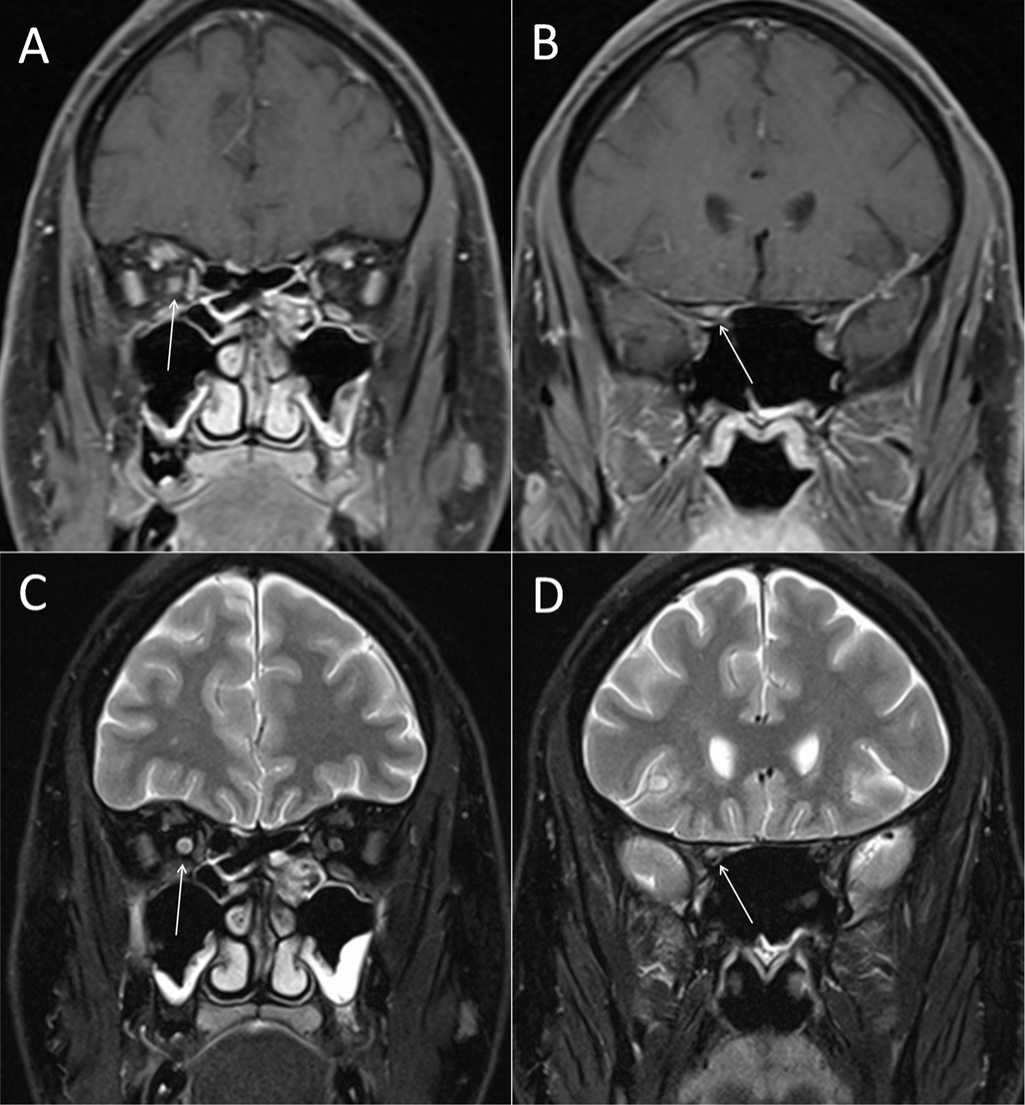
Gd enhancement and T2 lesion of the right optic nerve. Optic nerve MR imaging of a representative patient with ON. (A) Coronar fat-saturated T1-weighted MRI sequences of the intraorbital and (B) canalicular part of the optic nerve after application of 0.1mmol/kg gadolinium. The arrows highlight the Gd enhanced right optic nerve. (C) Signalalteration of the intraorbital and (D) canalicular part of the optic nerve in coronar fat-saturated T2 turbo spin-echo MRI sequences. The arrows highlight the hypertense T2 lesion of the right optic nerve.
